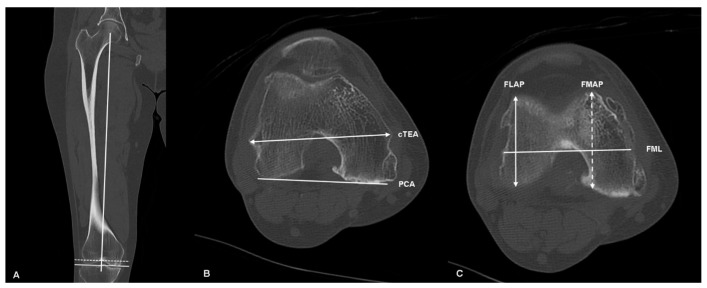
Radiographic measurements on computed tomography. (A) Determination of the distal femoral resection 9 mm proximal to the lowest point of the femoral condyle perpendicular to the mechanical axis of the femur. (B) The clinical transepicondylar axis and posterior condyle axis angles were drawn to assess distal femoral rotational alignment. (C) The anteroposterior length of the lateral femoral condyle (FLAP), the anteroposterior length of the medial femoral condyle (FMAP), and the mediolateral femoral length (FML) were measured.
